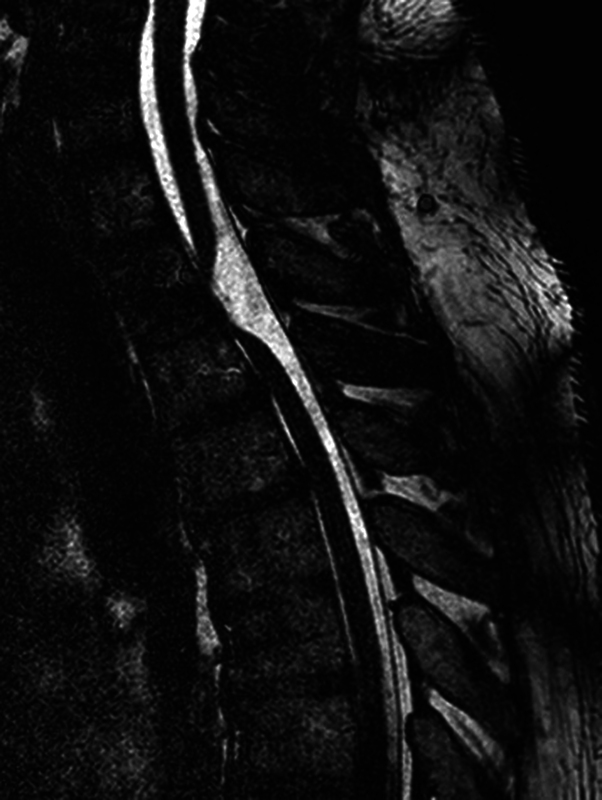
Magnetic resonance imaging (MRI) of the Spine – sagittal gradient echo.
